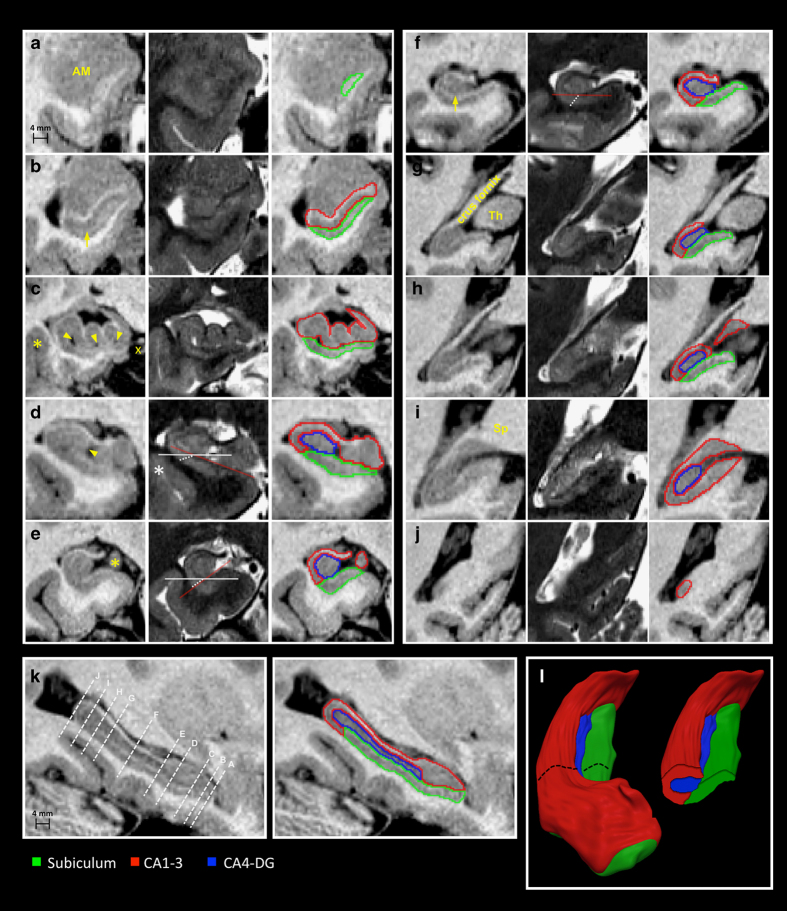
Anatomical boundaries of hippocampal subfileds on T1- and T2-weighted MRI. Sections displaying critical landmarks are shown. (a,j) are the most rostral and caudal coronal sections. (a) The rostral-most tip of the hippocampus is composed of the subiculum; at this level, the alveus surrounds the subiculum, separating it from the overlying amygdala (AM). (b) When CA1 first becomes visible, it runs parallel to the subiculum; the structures are separated by the subicular molecular layer (arrow). (c) Vertical digitations of CA1-3 (arrowheads point to cavities within the hippocampal sulcus; x indicates the ambient cistern); the supero-lateral subicular interface with CA1 is drawn along a line following the hippocampal sulcus, directed towards the fundus of the collateral sulcus (asterisk). (d) The rostral-most portion of CA4-DG is set at the section where the medial portion of the DG, the margo denticulatus, becomes visible (arrowhead). (e) Junction between head and body, at the level of the uncal apex (asterisk). (f) Hippocampal body; the arrow points to the molecular layer of the subiculum. (g) Rostral portion of hippocampal tail: the crus fornix is fully visible and well demarcated from the thalamus (Th). (h) The caudal slice of the subiculum is set to the posterior-most section on which the thalamus can be identified. (i) Middle segment of the tail. The subiculum is replaced by CA1-3, at the level at which the crus fornix fuses with the splenium (Sp) of the corpus callosum. (j) Terminal segment of the tail. (k) Sagittal hippocampal section displaying planes of the coronal cuts. (l) 3D surface rendering of hippocampal subfields with a coronal cut at the level of the body. On coronal sections, the orientation of the hippocampal body varies across individuals, modifying the spatial relationships between subiculum and CA1. In d, the hippocampus is oriented clockwise. In e, it is oriented counter-clockwise and in f it has a horizontal position. The red line follows the slope of the superior border of the subiculum, the solid white line represents the horizontal axis, and the dashed white line is placed at the boundary between subiculum and CA1.
